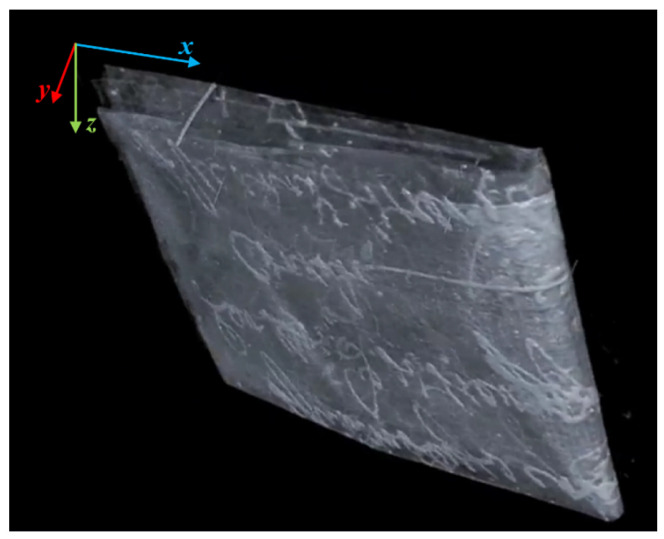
X-ray 3D tomographic reconstruction of the full folded document. The writings are clearly visible, but the page superimposition makes the document unreadable without ad hoc processing.
